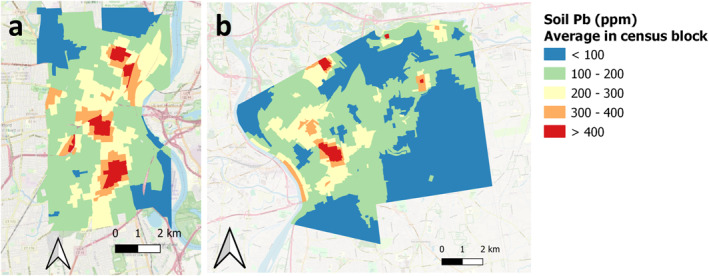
Distribution of Pb in census block soils extrapolated from individual samples published in Sirkovich et al. (2023) using ordinary kriging in panel (a) Hartford, CT and (b) Springfield, MA.
